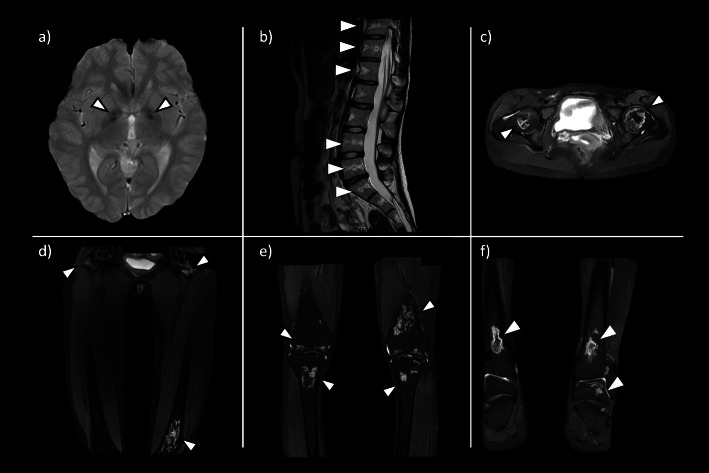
MRI (T2 weighted Image) findings were inconsistent with the patient’s age and revealed multiple sites of AVNSuspected calcification of the globus pallidus (a) and fatty changes in the lumbar spine (b: sagittal view), which are usually found in the elderly. AVN is seen in femoral head (c: axial view, d: coronal view); distal femur and proximal tibia (e: coronal view); distal tibia and talus (f: coronal view) AVN, avascular necrosis; MRI, magnetic resonance imaging
